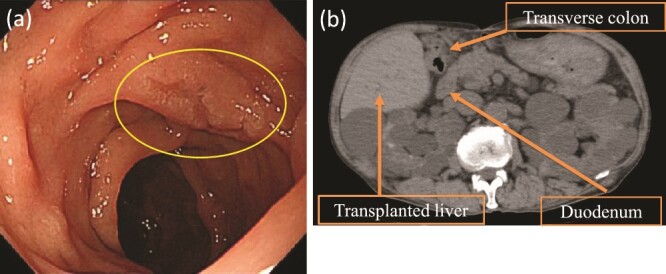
Upper gastrointestinal endoscopy and abdominal plain CT findings. (a) Upper gastrointestinal endoscopy showing the elevated lesion (10 mm in diameter) at the opposite side of the ampulla of Vater. (b) CT showing the transplanted liver very close to the transverse colon (axial image).
